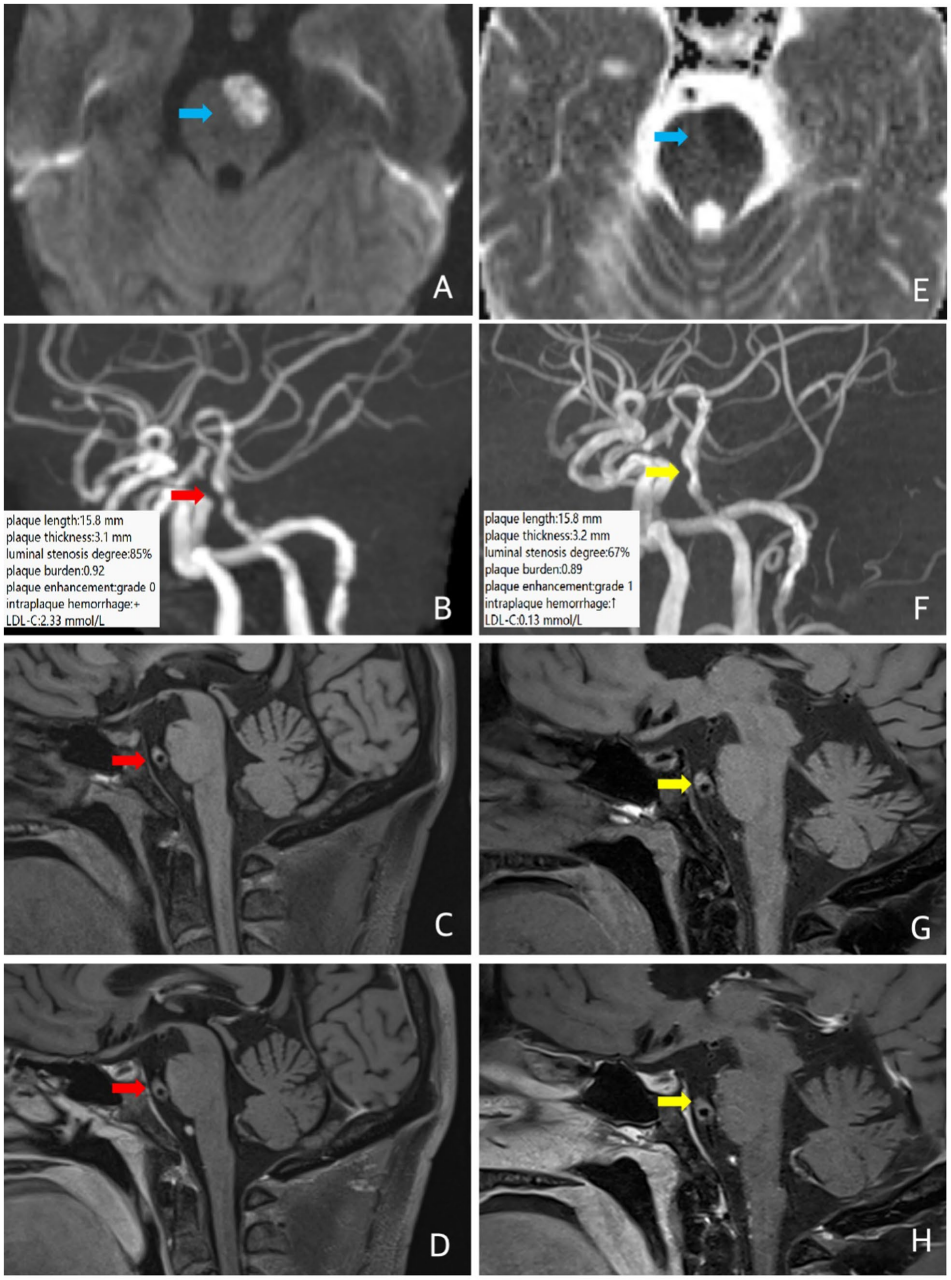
In case 4, patient at baseline (red arrow) and follow-up images 12 months later (yellow arrow). Three-dimensional time-of-flight cerebral magnetic resonance angiography showed the basal artery is tortuous and long, and the middle part is narrow. Coronal T1 plain scan weighted imaging (C,G) and enhanced imaging (D,H) in whole brain magnetic resonance imaging using different inversion angles to optimize contrast showed multiple atherosclerotic plaques were found in the basilar artery, especially in the middle segment, obvious stenosis of the lumen, eccentric thickening of the left wall of the basilar artery, and suspected intraplaque hemorrhage (T1 signal was higher than that of the temporal muscle nearby). There was no obvious enhancement of the plaque. The follow-up intracranial VW-MRI showed no significant changes in plaque length, plaque thickness, and plaque burden compared to baseline, a slight increase in luminal stenosis, plaque enhancement upgrades, and increased TI high signal (C,G), suggesting plaque progression. Which LDL-C level decreased significantly. In case 4, diffusion sequence (blue arrow) showed limited diffusion in the left anterior portion of the pons, increased signal on DWI (A) and decreased signal on ADC (E), suggesting a fresh infarct.
